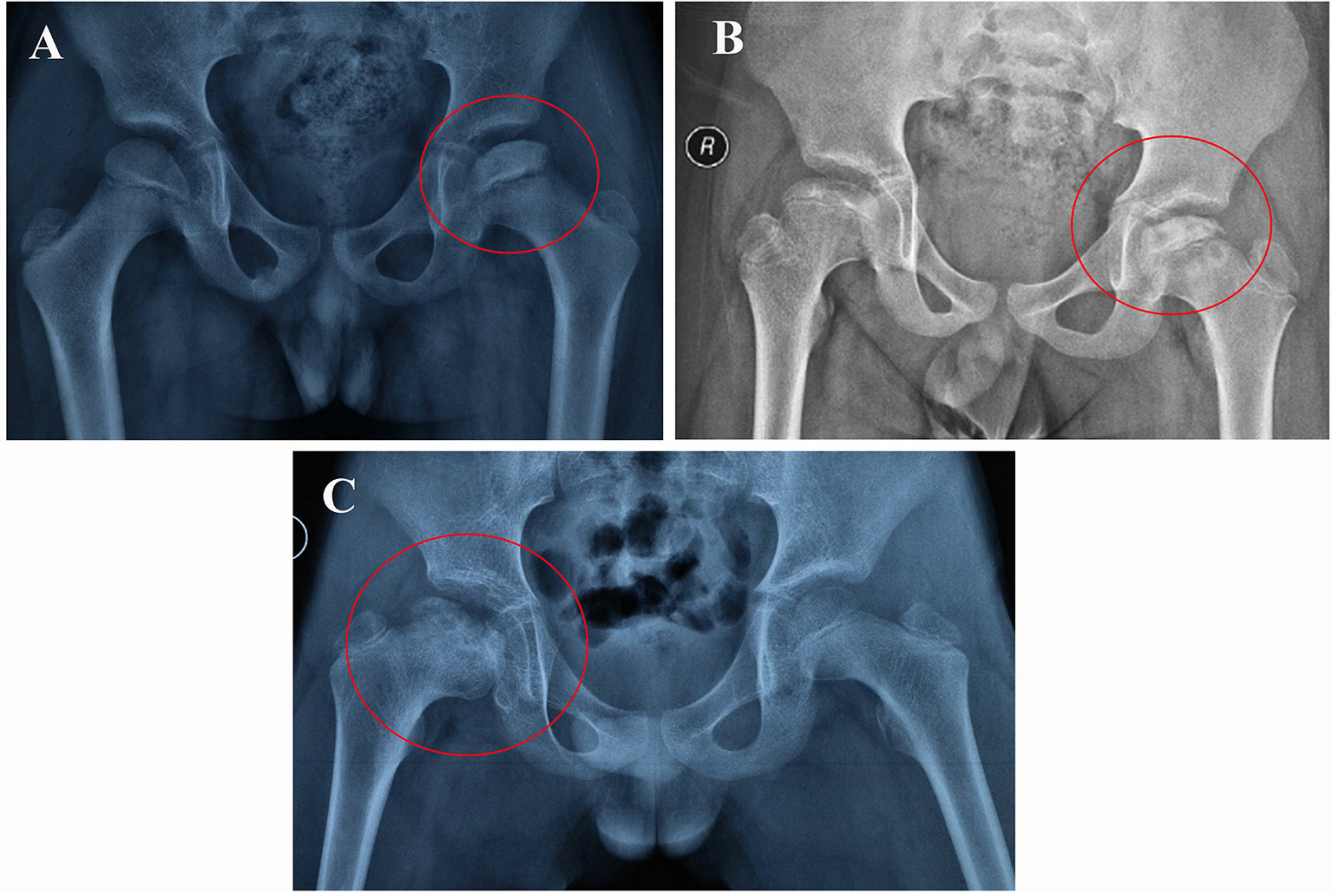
The plain radiographic appearances of the femoral head from different stages of LCPD patients (the red circle). Necrosis stage, 8-year old boy involved left femoral head (A); Fragmentation stage, 9-year old boy involved both hip (B); Reossification stage, 10-year old boy involved right hip (C).
